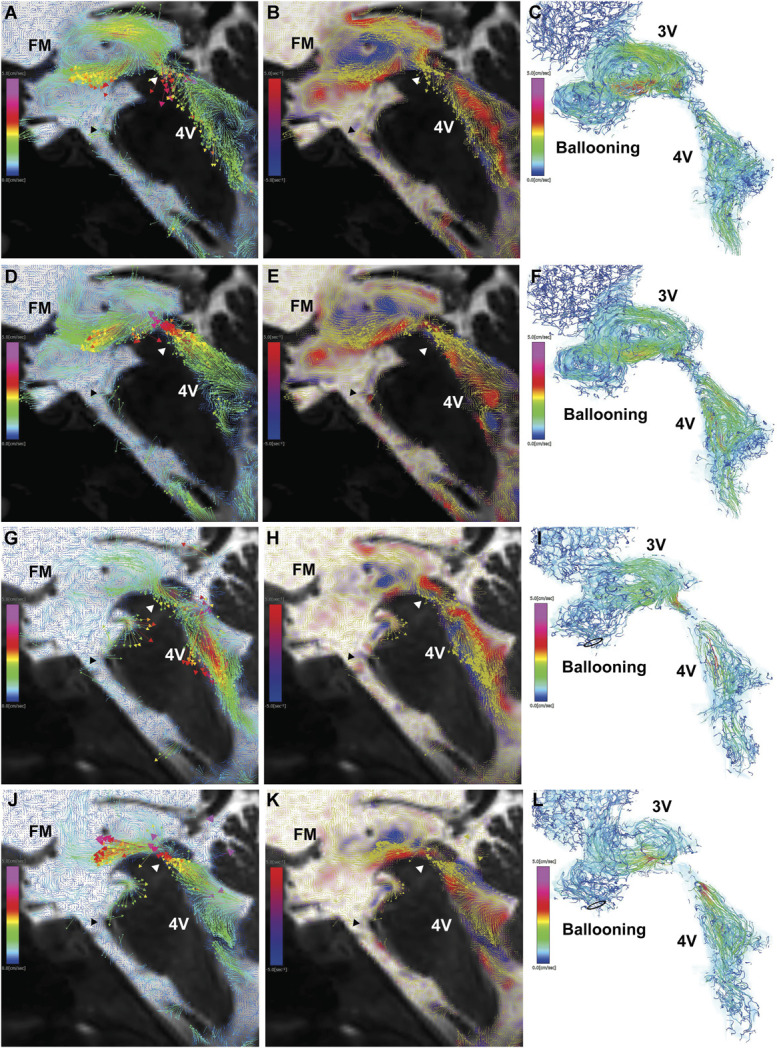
Communicating hydrocephalus (case 4). A-F, 4D flow MRI before VPS. G-L, 4D flow MRI after VPS. A, D, and G, Velocity maps of 2D sagittal views, with colored arrows indicating velocity vectors. B, E, and H, Vorticity maps of 2D sagittal views, showing clockwise vortices in blue, counterclockwise vortices in red, and overlaid yellow velocity vectors. C, F, and I, Streamlines of CSF motion during a heartbeat in the 3D view, white arrowhead, upper end of the cerebral aqueduct. Rapid CSF flow from the cerebral aqueduct into the 4V and reversed rapid CSF flow from the cerebral aqueduct into the 3V were observed. A clockwise vortex formed in the posterior 3V, whereas a counterclockwise vortex developed in the anterior 3V with a downward-protruding floor (black arrowhead). After VPS, flow velocity from the cerebral aqueduct into the 4V increased, whereas flow velocity from the cerebral aqueduct into the 3V decreased compared with preshunting imaging. Although ballooning of the 3V floor persisted, the counterclockwise vortex in the anterior 3V had nearly disappeared. 2D, 2-dimensional; 3D, 3-dimensional; 3V, third ventricle; 4D, 4-dimensional; 4V, fourth ventricle; CSF, cerebrospinal fluid; FM, foramen of Monro; VPS, ventriculoperitoneal shunting.
